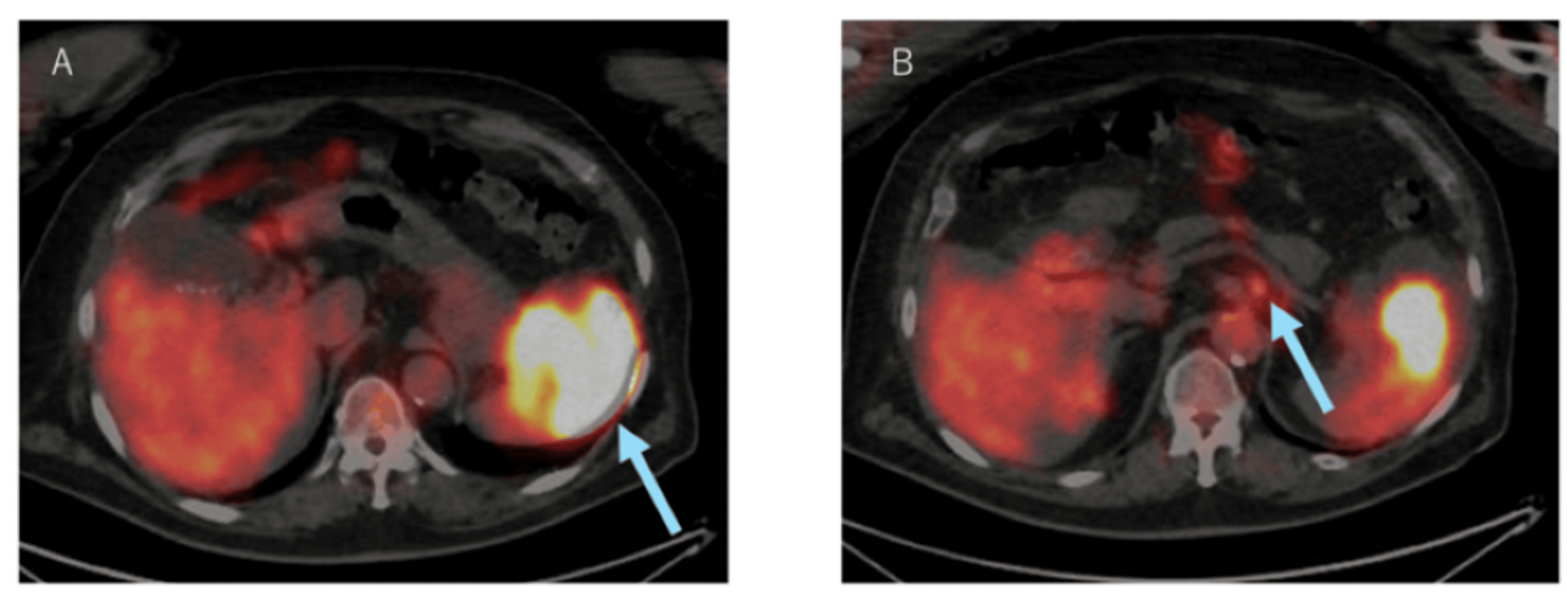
Positron emission tomography/computed tomography (PET/CT) Fused PET/CT images show intense fluorodeoxyglucose uptake in both the splenic tumor (A) and the perisplenic artery lymph node (B). The maximum standardized uptake values were 20.4 and 3.7, respectively.
